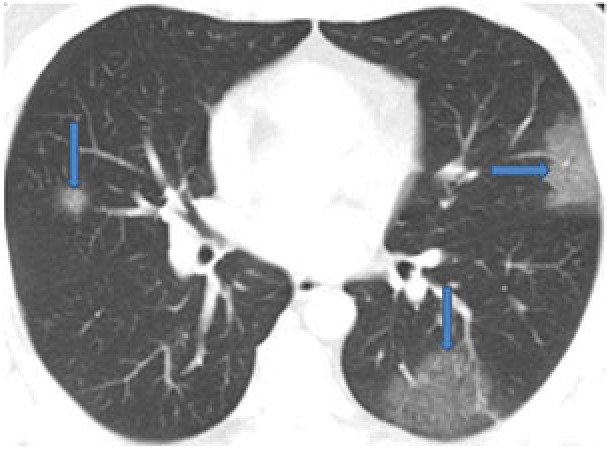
A 35-year-old male COVID-19 patient presenting fever and headache for four days. CT scan shows pure ground-glass opacities in bilateral multilobe (arrows).
